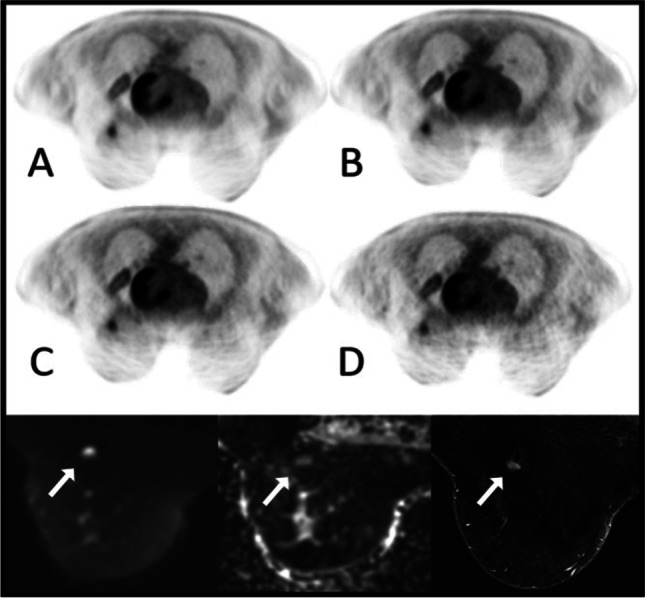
PET and MRI images of a 57-year-old breast cancer patient with an increase of image noise from 20 (A), 15 (B), 10 (C) to 5 min (D) and an associated slight subjective image quality reduction from 20/15/10 towards 5 min (image quality 3 vs. 2 according to the 5-point scale). The fast breast MRI protocol (white arrow: primary) visualized in the lower section (left: DWI, middle: ADC, right: T1-early CE-2) provides adequate diagnostic information
